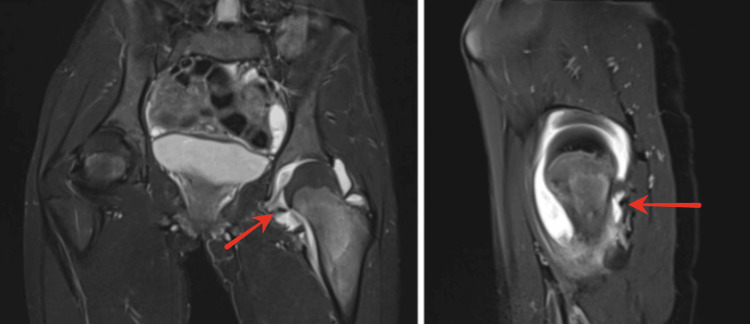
Coronal and Sagittal MRI Images Showing Bone Marrow Edema Surrounding Osteoid Osteoma Coronal and sagittal MRI images show extensive bone marrow and periarticular edema involving the femoral neck and adjacent hip joint structures, without evidence of epiphyseal displacement. MRI, magnetic resonance imaging.
